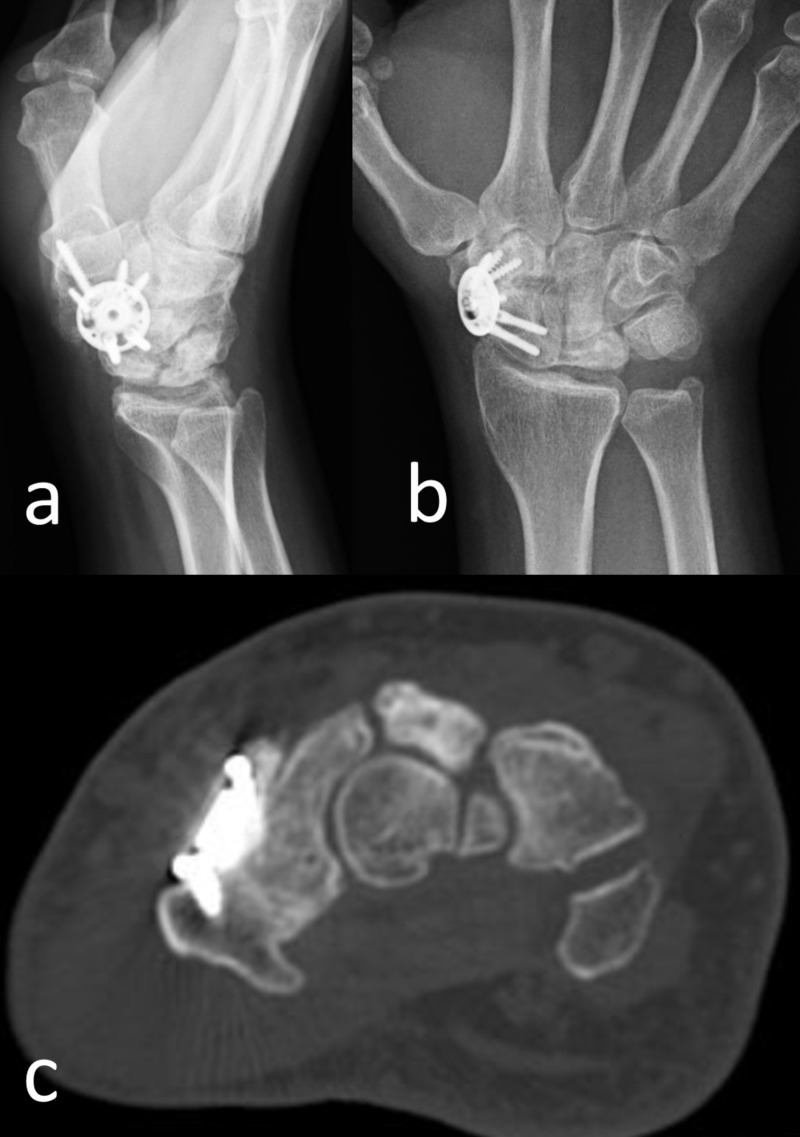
Postoperative Imaging of Case 4 A) Lateral and B) anteroposterior wrist radiographs at the final follow-up at the 18th postoperative month. C) computed tomography examination demonstrated complete union and consolidation of the arthrodesis.
